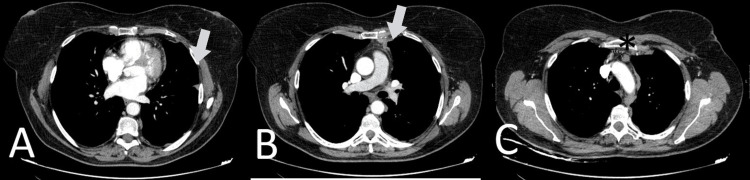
Computed tomography scan (June 2022) - with significant reduction of pleural thickness (arrows), and 11 mm adenomegaly (*)
